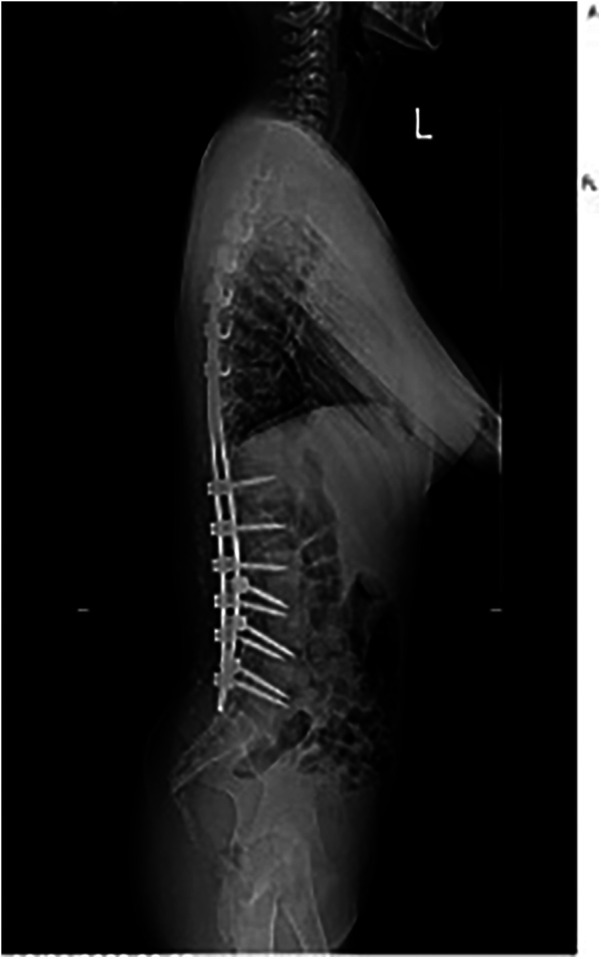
Postoperative lateral radiograph demonstrating sagittal plane alignment following posterior spinal fusion.
